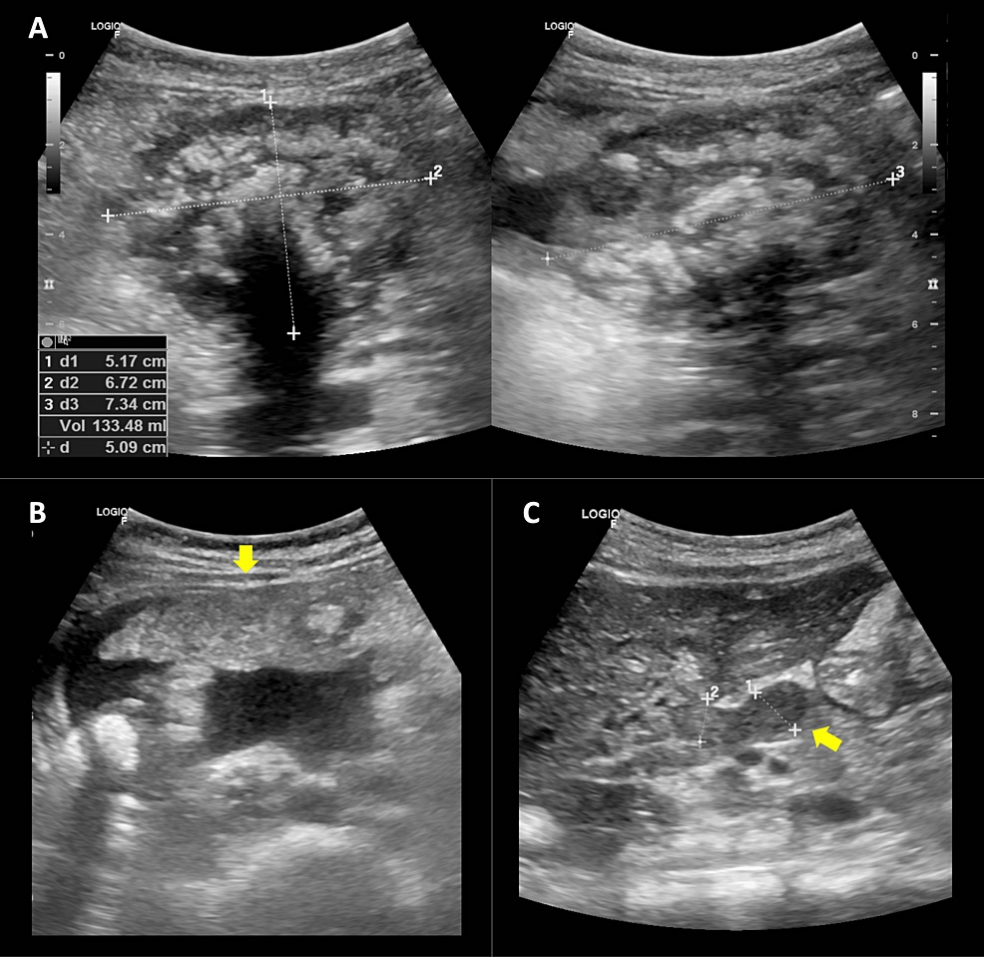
Ultrasound A, located in the mesogastrium, the dominant mass appears heterogeneous with poorly defined edges and some hyperechoic areas causing a posterior acoustic shadow, measuring up to 7.3 cm; B, thickening and increased echogenicity of the omentum (arrow) associated with moderate ascites; C, periportal lymphadenopathy of up to 14 mm (arrow)
